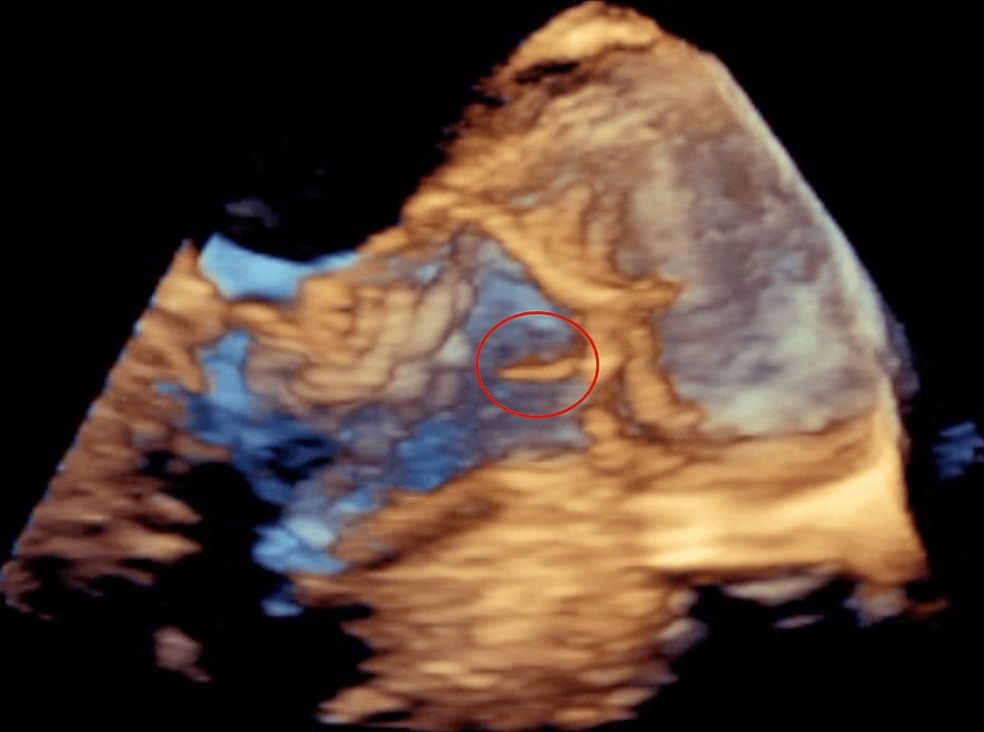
3 dimensional transesophageal echocardiography showing sclerotic aortic valve and an echogenic, highly mobile structure (red circle) attached to the ventricular surface of the right coronary aortic valve cusp measuring 0.8 x 0.25 cm.
